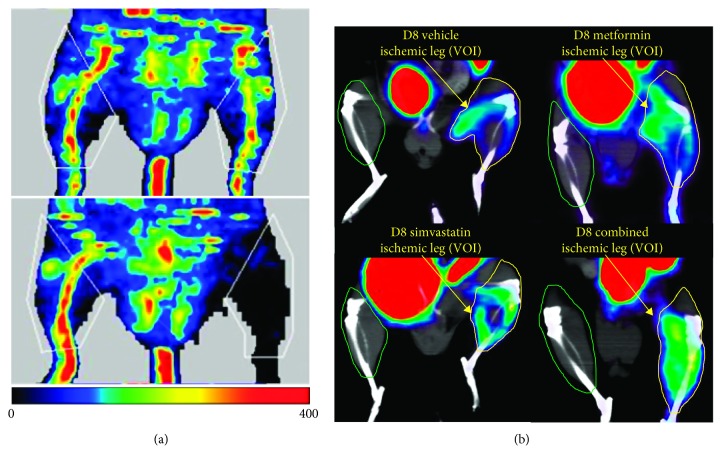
Representative images displaying (a) laser Doppler perfusion imaging assessment of tissue perfusion before (upper panel) and after femoral artery ligation (lower panel). The region of interest placement is indicated in white. (b) Representative images showing uptake of [^18^F]FtRGD in the ischemic leg at day 8 in each of the diabetic treatment groups (representative nondiabetic image not shown). The region of interest placement for the ischemic leg is indicated in yellow, and region of interest placement for the contralateral intact leg is shown in green.
